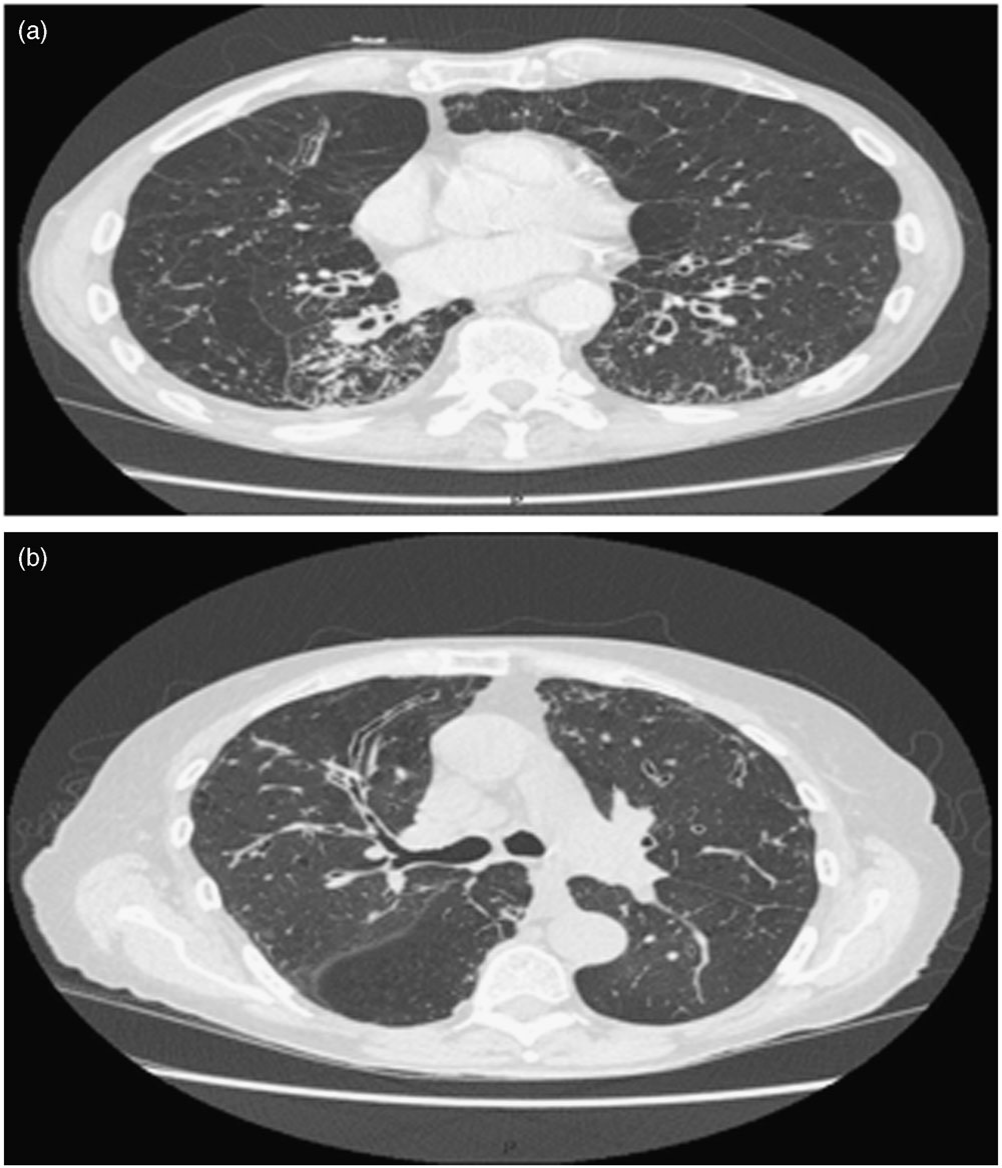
Example high-resolution chest computed tomography images of bronchiectasis. a Cylindrical bronchiectasis; b longitudinal or varicose bronchiectasis
